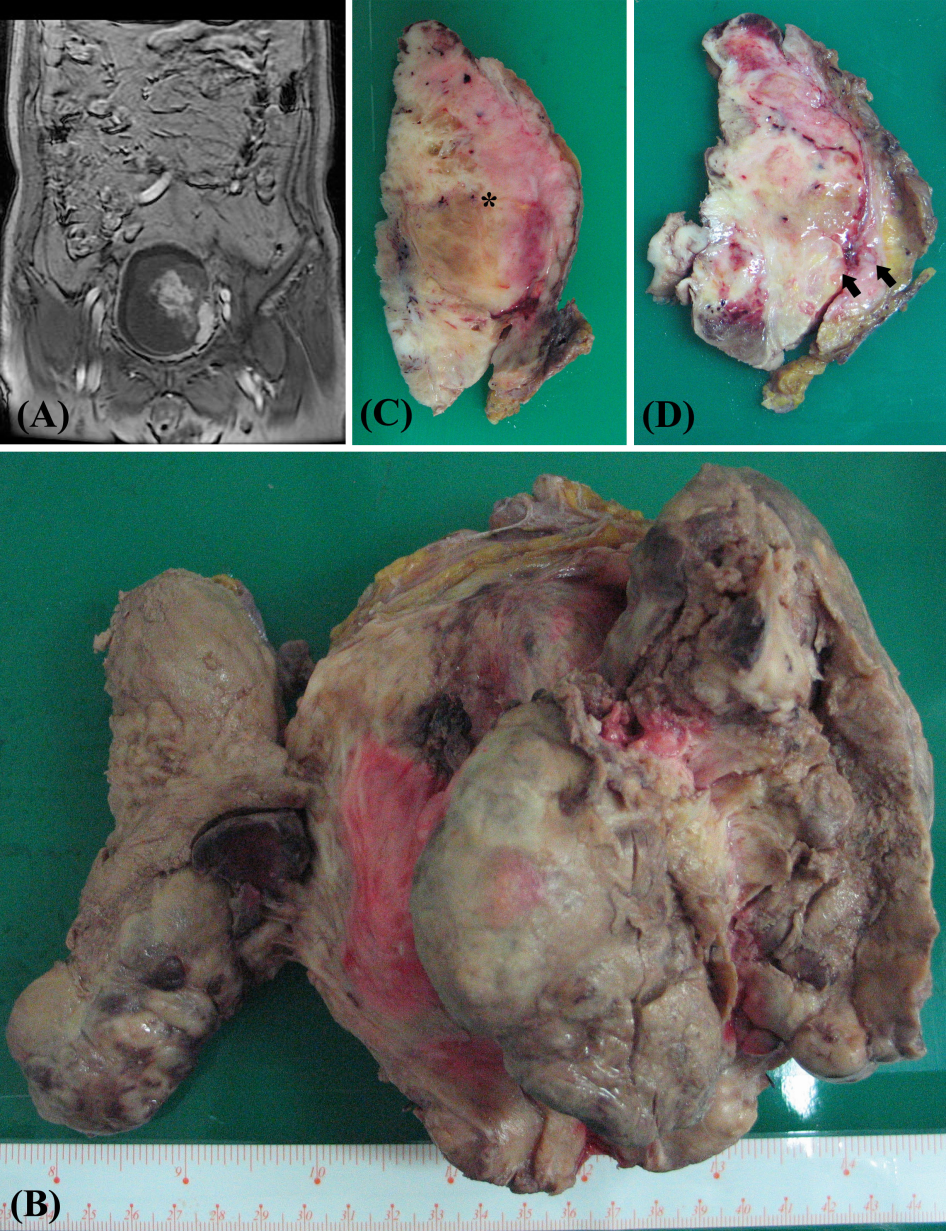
(A) Magnetic resonance imaging (MRI) of the pelvis demonstrated a large mass in the urinary bladder that shows focal contrast medium enhancement on the left side with adjacent vesical wall thickening and obliteration of the lower signal intensity on T2WI. (B) Macroscopically, the urinary bladder contained a nonencapsulated, huge, and lobular mass, which was located in the left trigone and measured 14 × 13 × 7 cm in size. (C) and (D) On the cut surface, this tumor was composed of a predominant white, myofibrous-like component, and myxomatous (asterisk), hemorrhage, and tumor necrosis foci. Several hard osteochondroid-like nodules were also palpable within this tumor (arrow).
